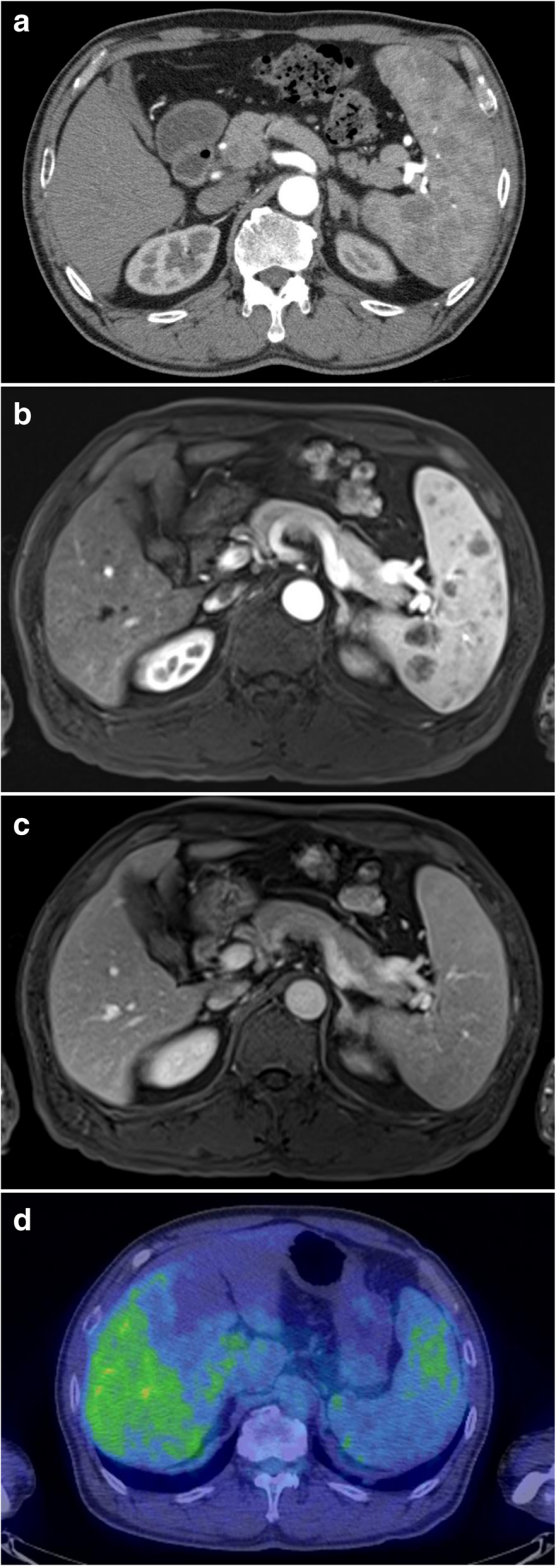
a Contrast-enhanced computed tomography findings. Multiple mass lesions of low density are noted in the arterial phase. b Findings of dynamic magnetic resonance imaging in the early arterial phase. The mass lesions show low signal intensities. c Findings of dynamic magnetic resonance imaging in the equilibrium phase. The mass lesions show signal isointensities. d Positron-emission tomography computed tomography findings. Image shows splenomegaly without an abnormal uptake
